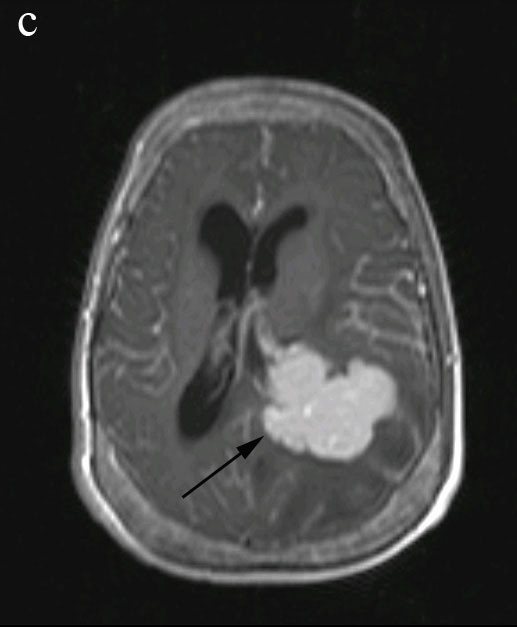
Axial T1-weighted MRI image with IV contrast demonstrating a large homogenously enhanced lesion in the left trigone area.
